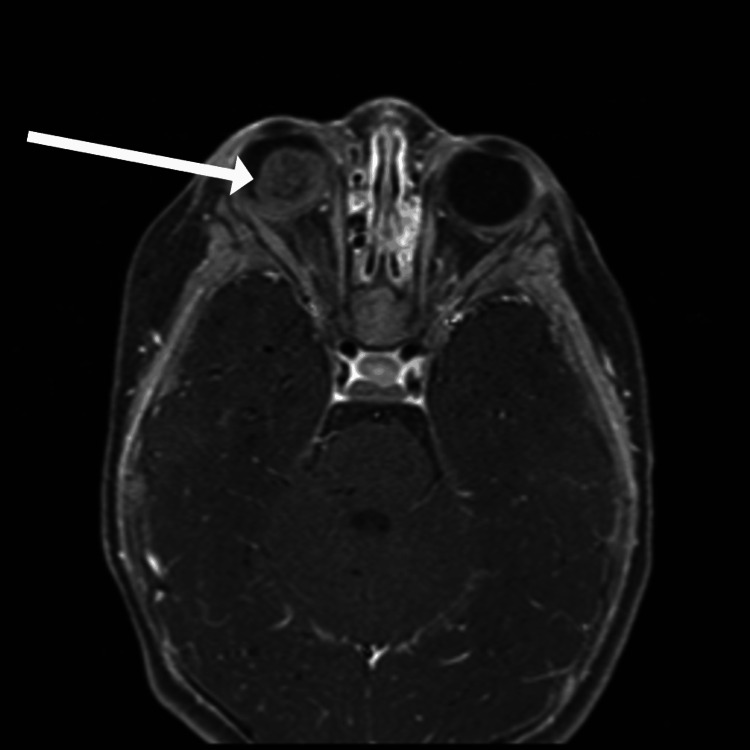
Axial view of MRI of the orbits during initial hospital stay. White arrow indicates intraocular mass within the right globe with internal restricted diffusion and microhemorrhage, consistent with retinoblastoma.
